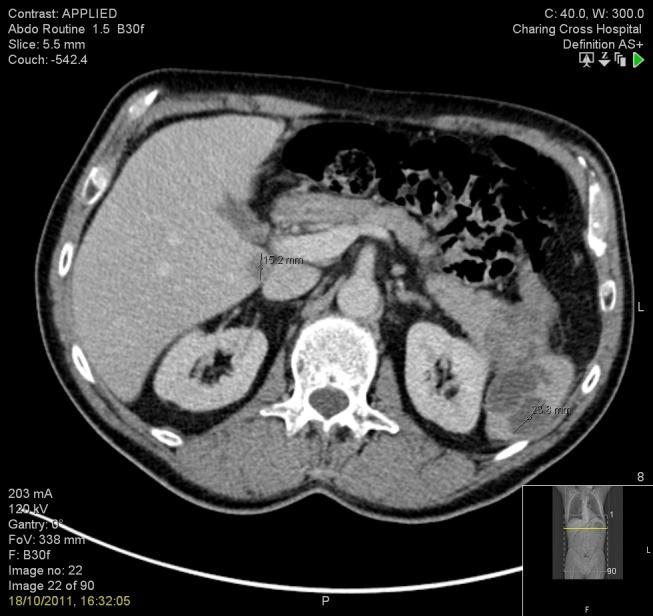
CT showing liver metastasis in segment VI
